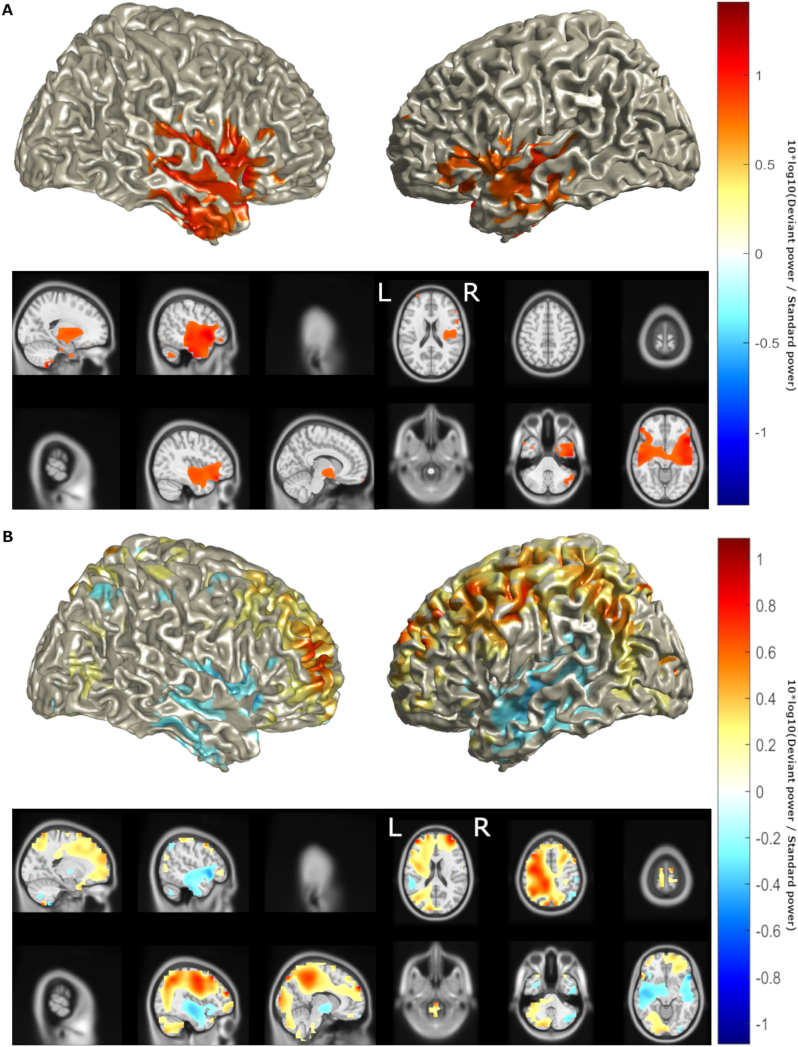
LCMV identified a pattern of decreased activity in bilateral superior temporal and inferior frontal sources, and an increase in activity in the left hemisphere. Location of MMN sources with (a) top 25% of power (10*log_10_(Deviant power / Standard power)) in healthy controls and (b) power differences >25% of maximum between ALS patients and healthy controls as determined by LCMV beamforming. Red denotes increase in power, blue denotes decrease in power. Axial MRI views are from above (L-Left, R-Right).
